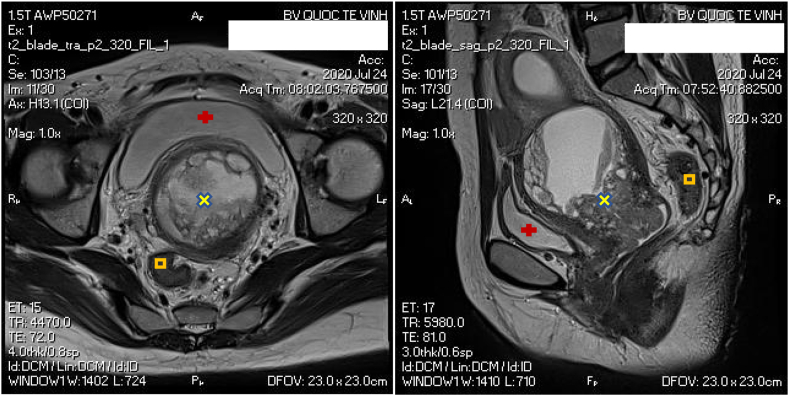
Pelvic magnetic resonance image showing an enlargement of the cervix with pelvic lymph nodes of about 8 × 9mm in size and a slightly enlarged uterus with a fluid filled uterine corpus (about 51–70mm). The cervical parametrium is intact. (x: the tumor, +: bladder, □: rectum).
